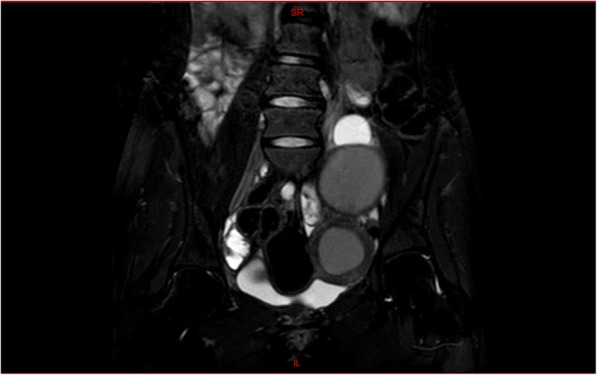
Pelvic magnetic resonance imaging: T2 signal magnetic resonance imaging indicated the presence of left endometrioma above the hematometra
